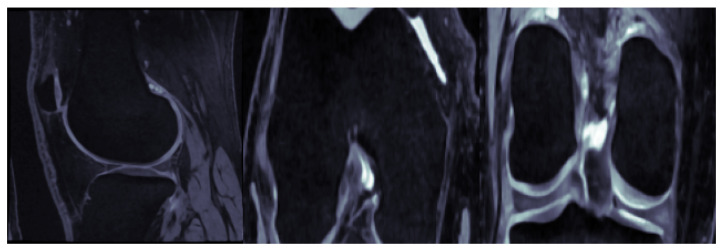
A typical knee MRI viewed in three orthogonal planes (left to right: sagittal, coronal, axial).
